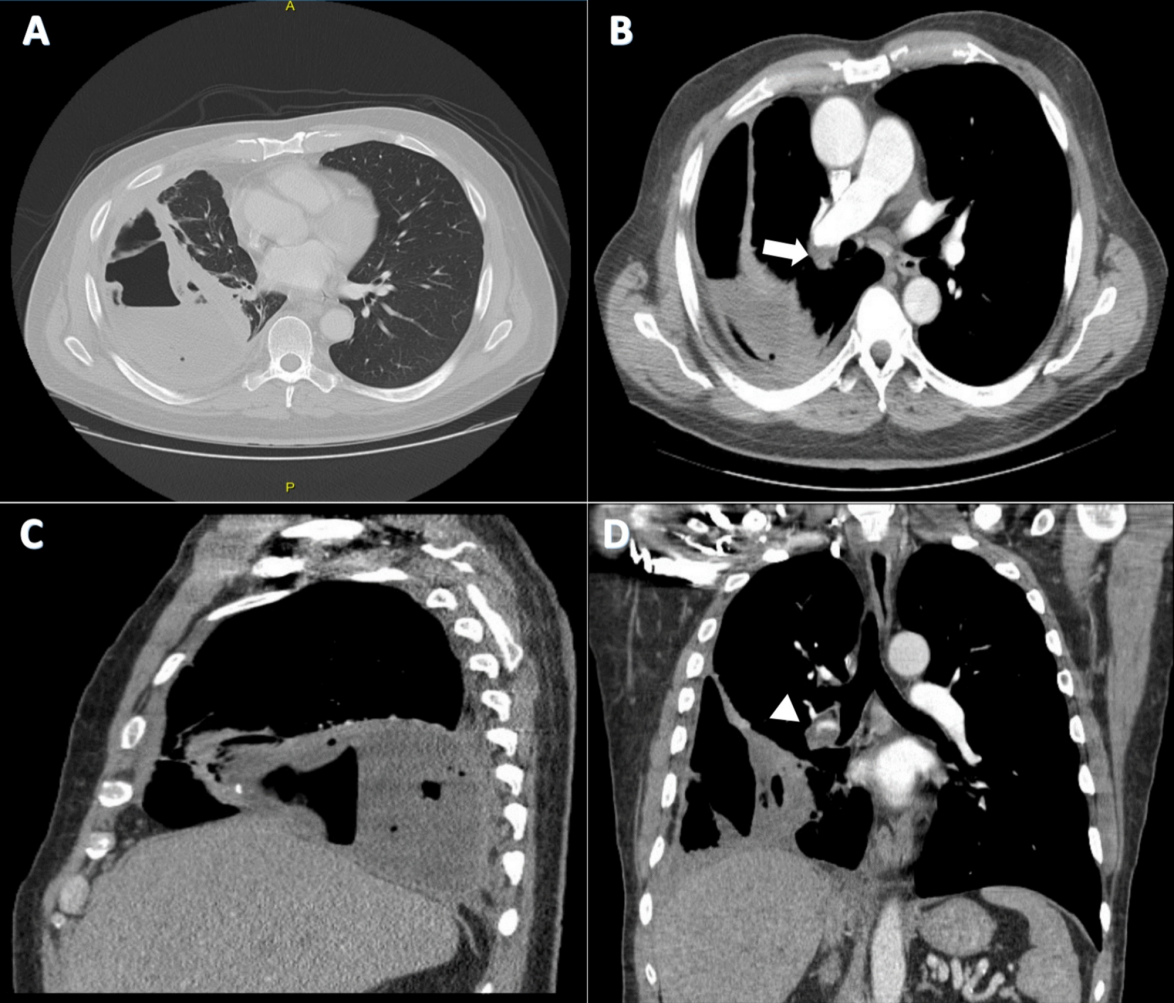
Chest CT with contrast with sagittal and coronal reconstruction views. (A) Axial lung window reveals walled-off collection with air-fluid level in the right lower chest. (B) Distal right main pulmonary artery occluded by pulmonary embolus can be seen (arrow). Sagittal view (C) highlights the collection described above and coronal view (D) highlights the collection as well as the pulmonary embolus in the right pulmonary artery (triangle).
